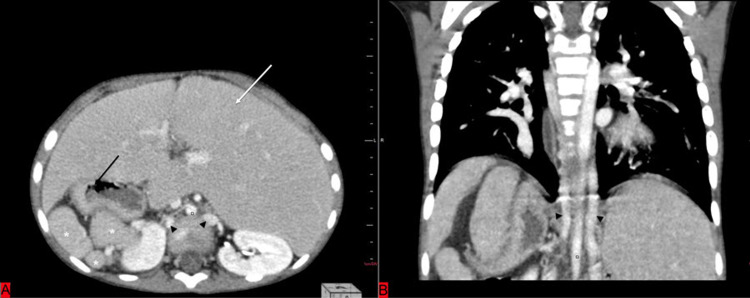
Reconstructed axial (A) and coronal (B) sections of abdominal CT of a five-year-old child suffering from heterotaxy syndrome revealing an enlarged liver (white arrow), multiple small-sized spleens (asterisk), fundus of stomach present on right side (black arrow), a malpositioned right kidney, and two hemiazygos veins (black arrowheads).
